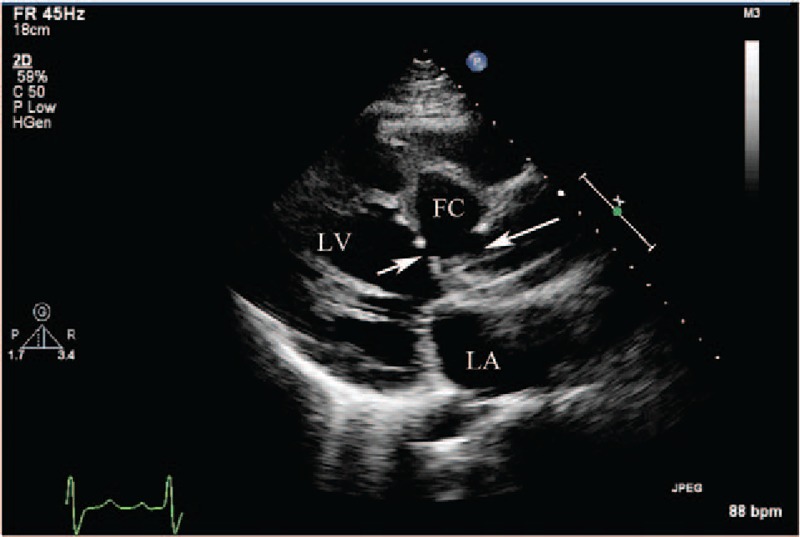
(Patient #11) Transthoracic echocardiogram (parasternal long axis view) showing a mechanical aortic valve in situ with annular detachment and the entry site of rupture (arrow on right) from the right sinus of Valsalva aneurysm into the dissecting false interventricular septal cavity, and the entry site of rupture (arrow on left) from the false interventricular septal dissecting cavity into the left ventricular cavity. (See also video 3). FC = false cavity, LA = left atrium, LV = left ventricle.
